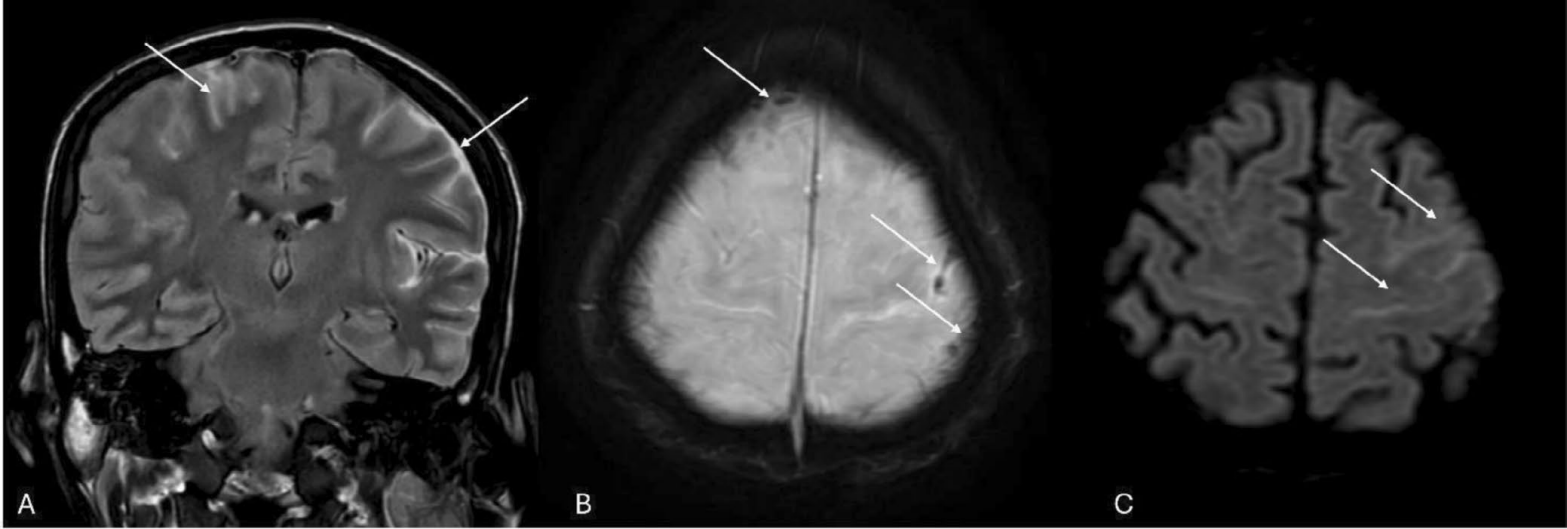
MR images of the brain. (A) FLAIR coronal post‐contrast image showing diffuse sulcal hyperintensity, more on the left side (arrows). (B) Susceptibility weighted image shows areas of dropout in the sulcal spaces (arrows), likely attributable to paramagnetic properties of fungal elements and arachnoiditis. (C) Subtle corresponding diffusivity also noted on diffusion‐weighted axial image (arrow).
